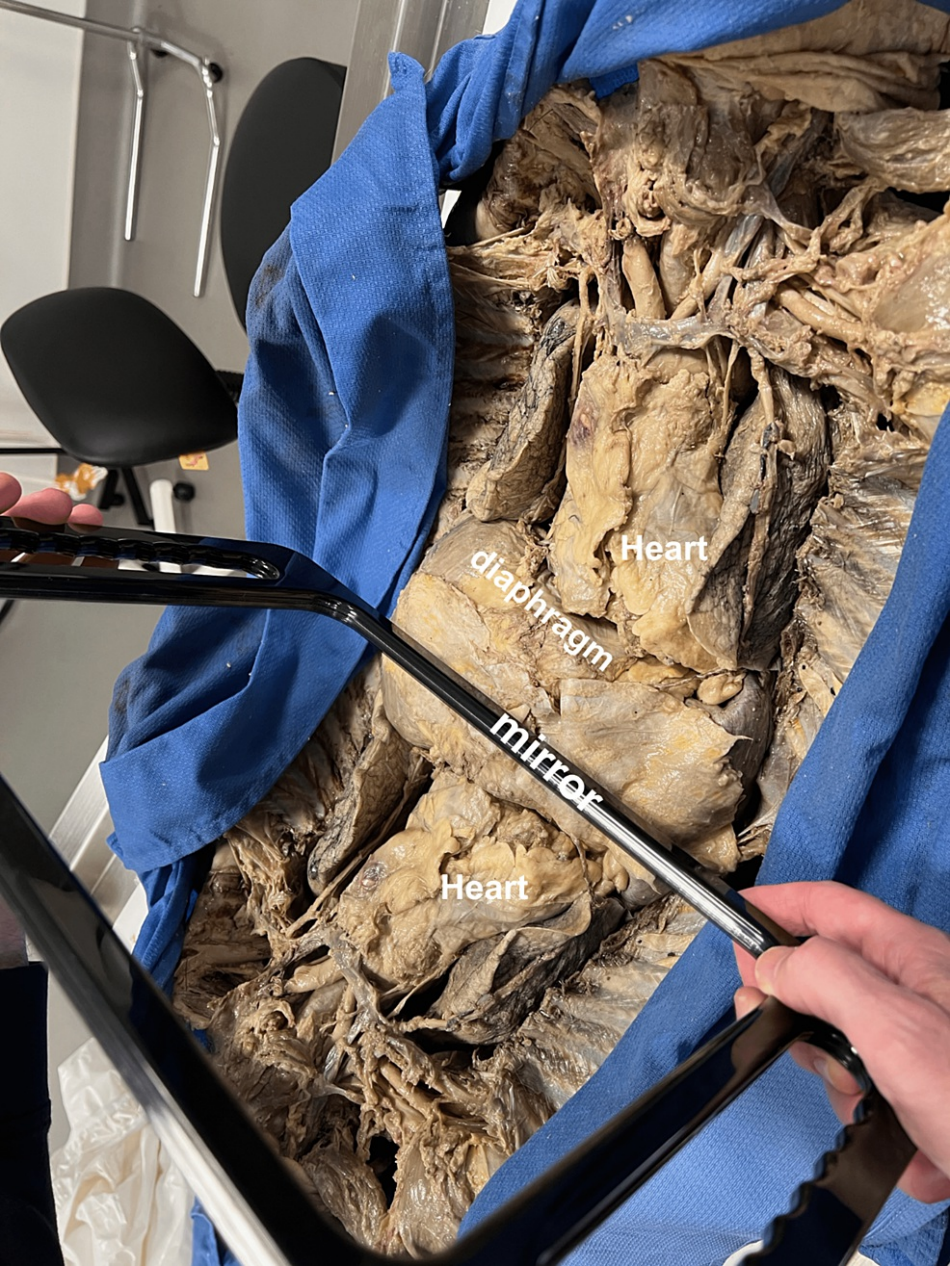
Subxiphoid mirror A mirror held in the position of the probe portrayed a reflection of the heart in its natural position, mimicking the view on the ultrasound screen.
